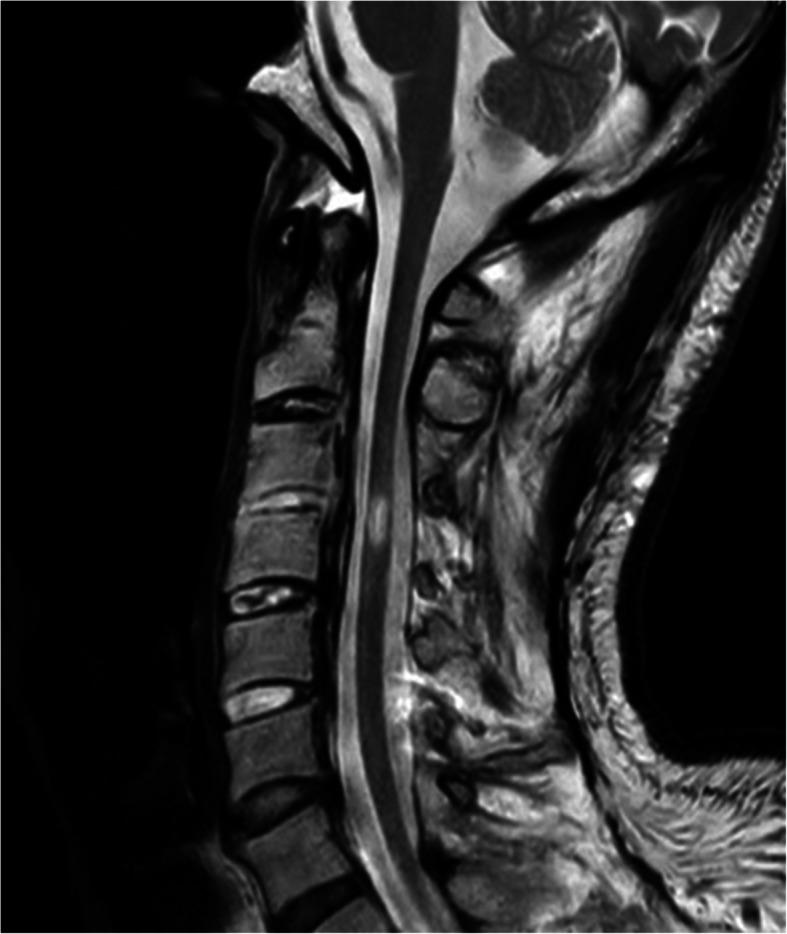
A cervical spine sagittal T2-weighted magnetic resonance image showing a C3-C4 spinal cord lesion
